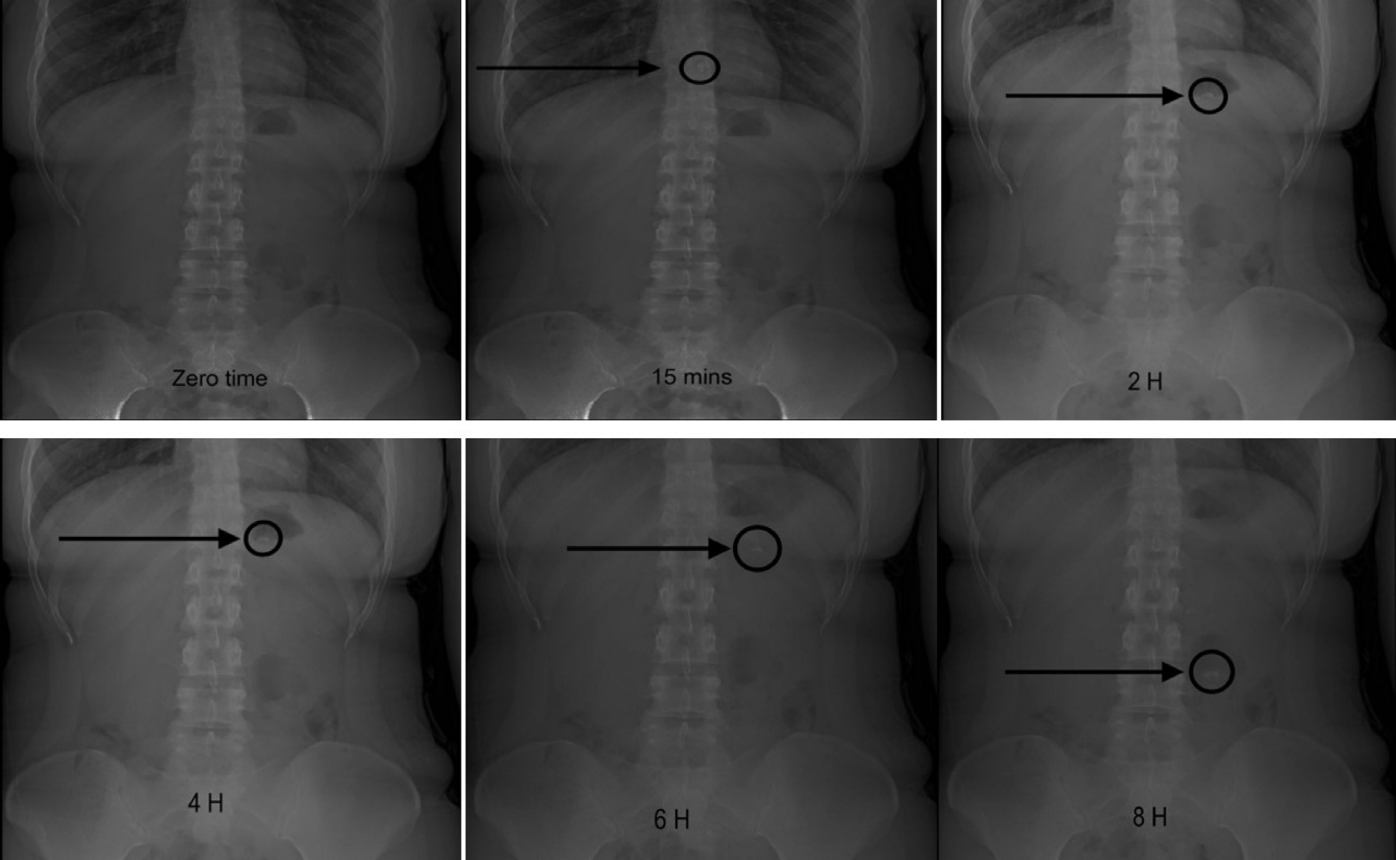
X-ray images of the ORS (BaSO_4_-loaded) representing its location in the abdomen of a human volunteer at different time intervals (hours). Note: The location of the ORS is represented with an arrow.
